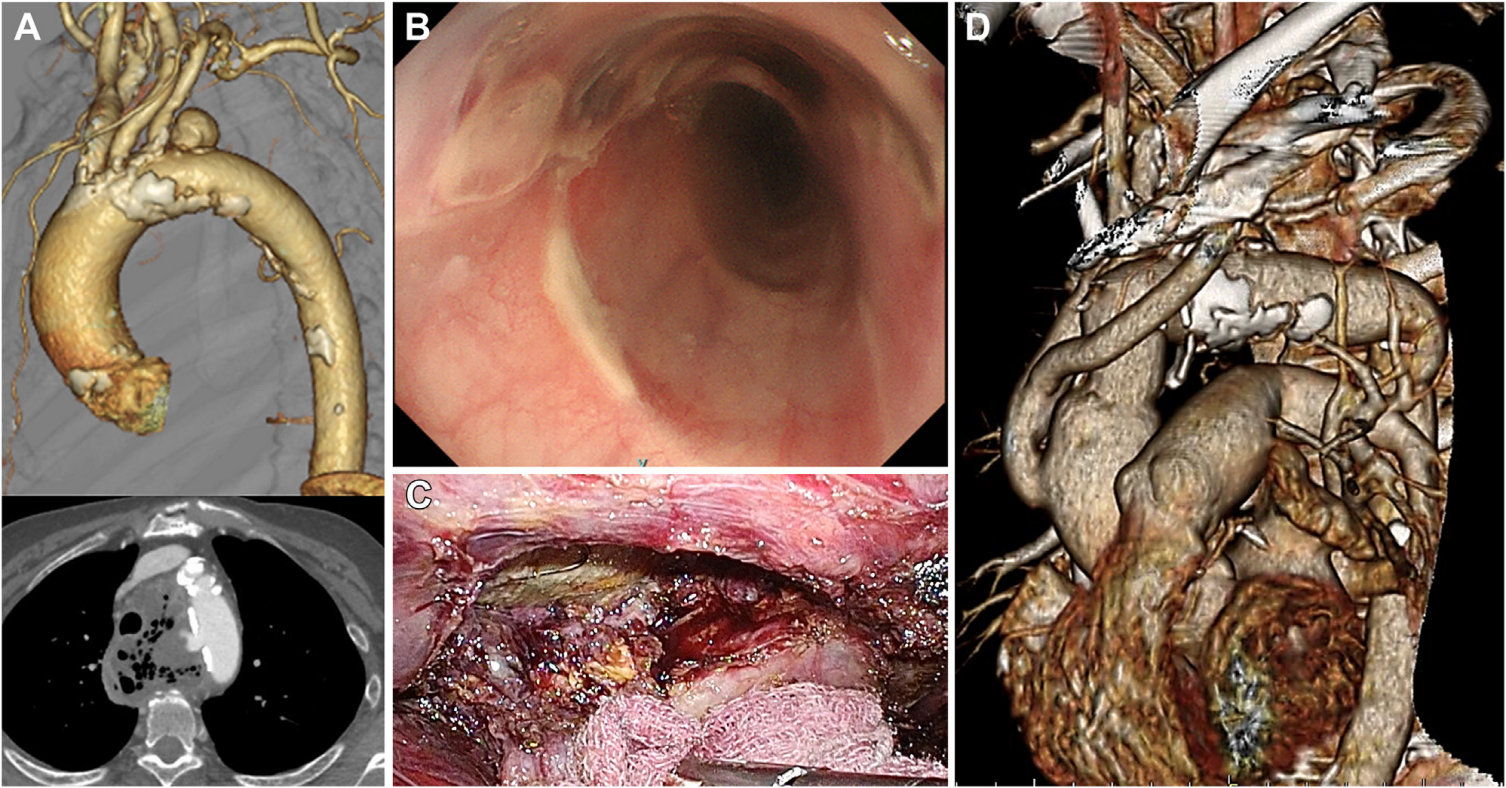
(A) Preoperative computed tomography angiography images. (B) Gastroesophageal endoscopic findings. (C) Intraoperative view during video-assisted esophagectomy. (D) Postoperative computed tomography angiography image.
